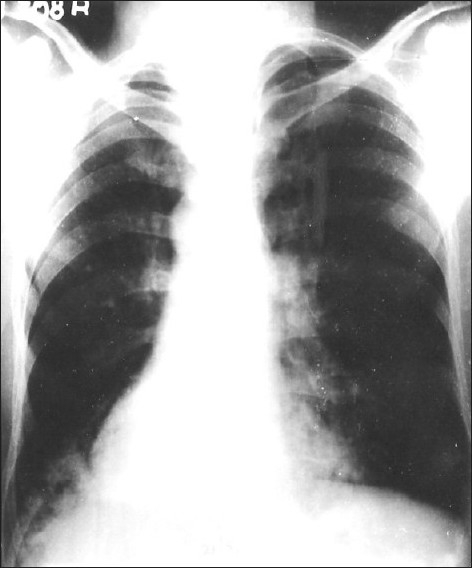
X-ray chest, PA view, showing dextrocardia and inhomogeneous infiltrate at both the lower zones in paracardiac region
